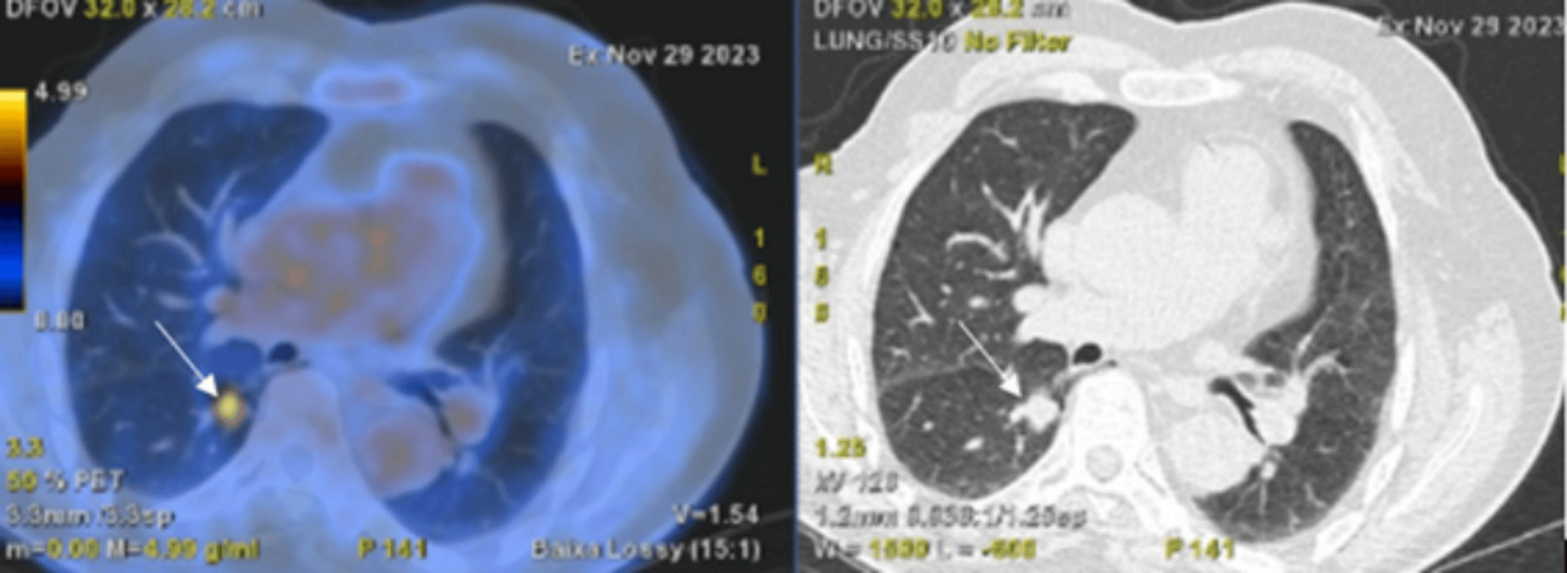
PET/CT scan of the thorax 18F-fluorodeoxyglucose positron emission tomography/computed tomography (18F-FDG PET/CT) scan of the thorax showing increased metabolic activity within the pulmonary nodule (white arrow), highly suggestive of malignancy.
